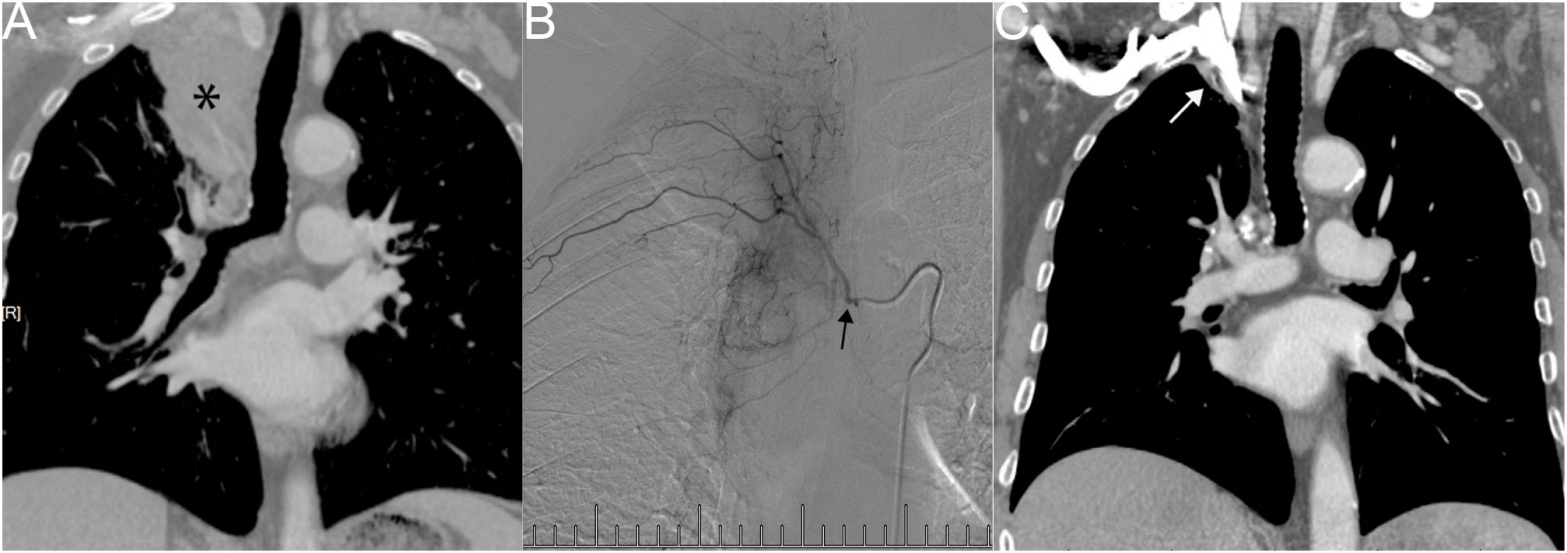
Male, 83 years old, with a right upper lung tumor, discovered due to chest pain, confirmed by biopsy as squamous cell carcinoma (T4N3M0). (A) CT Coronal plane image showing right upper lung tumor with atelectasis of the right upper lobe, hilar, and mediastinal lymph node metastasis (*). (B) A right bronchial artery angiography indicating blood supply to the right lung tumor, which can be perfused with chemotherapy and embolization (→). (C) CT Coronal plane image after six courses of BACE, demonstrating that the right upper lung tumor and atelectasis have basically disappeared (→).
